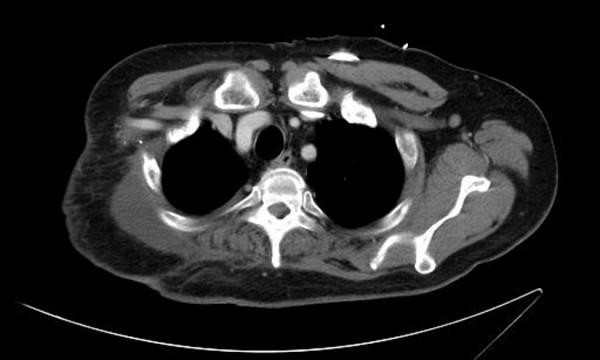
Post-operative CT scan showing the intact chest wall and removal of tumor.
